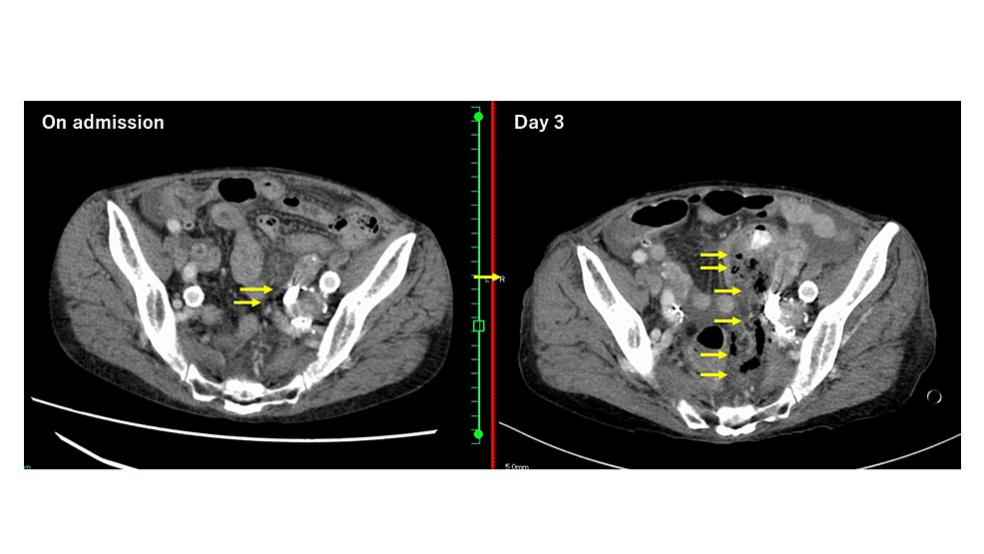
Comparison of abdominal contrast-enhanced CT scan on admission with that on day 3 Images that were taken on admission show a small amount of free air in the mesentery. The day 3 image shows a discontinuity in the sigmoid colon wall and free air and bowel contents in the surrounding mesentery.
